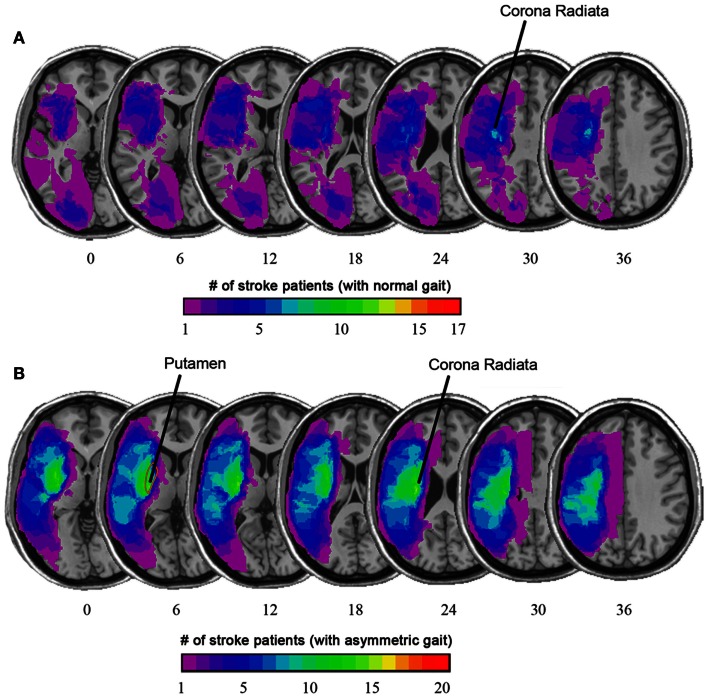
(A) Overlaid on the average T1-weighted image is a color map showing the average lesion location as a proportion of stroke patients with normal gait. (B) Among stroke patients with asymmetric gait, there was a higher prevalence of stroke lesion that included the putamen and corona radiata. (Based on Alexander et al., 2009).
